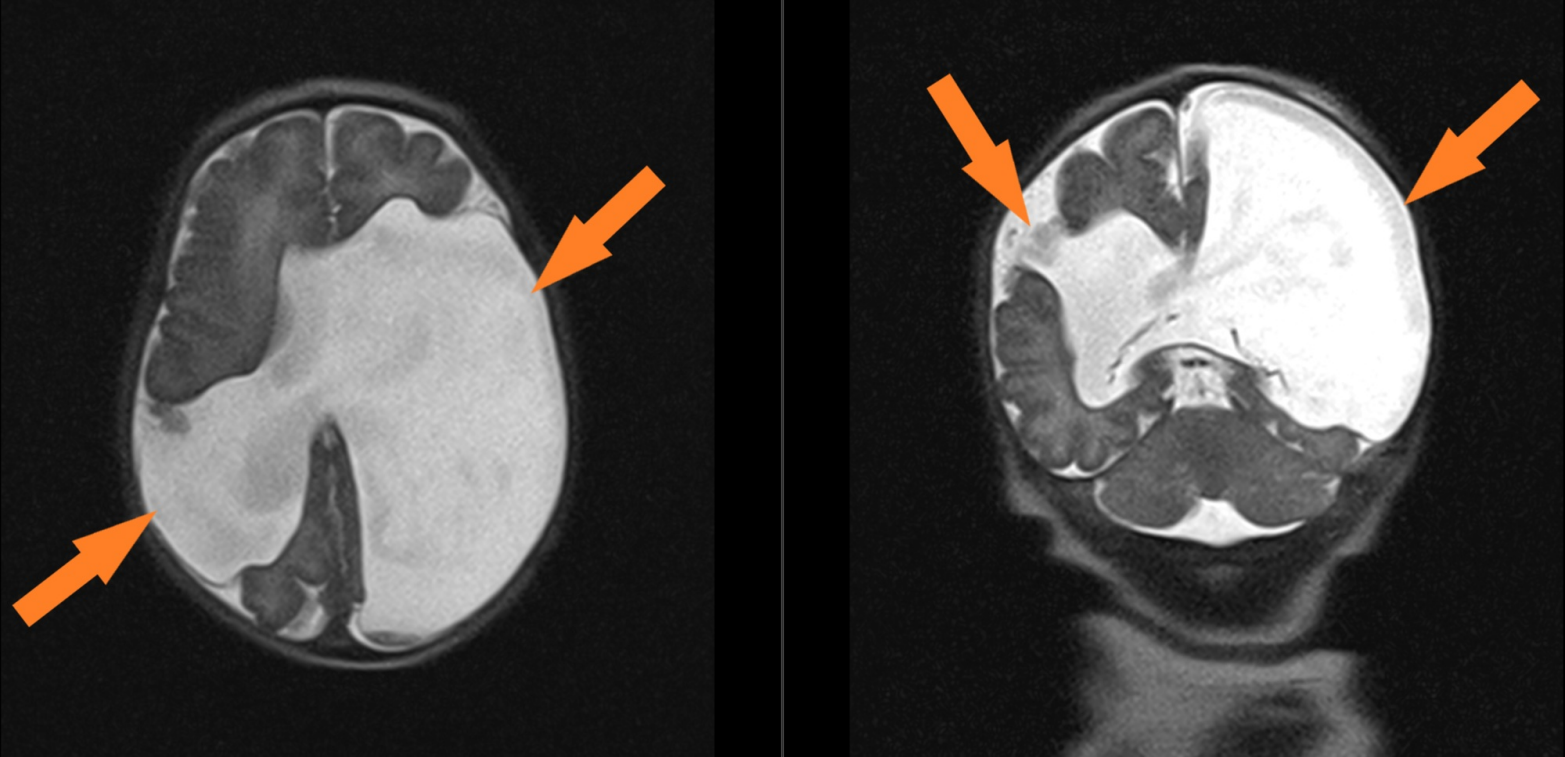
Neonatal MRI at day of life 1 Images from patient’s brain MRI at day of life 1 showing porencephaly in the bilateral middle cerebral artery territories.
